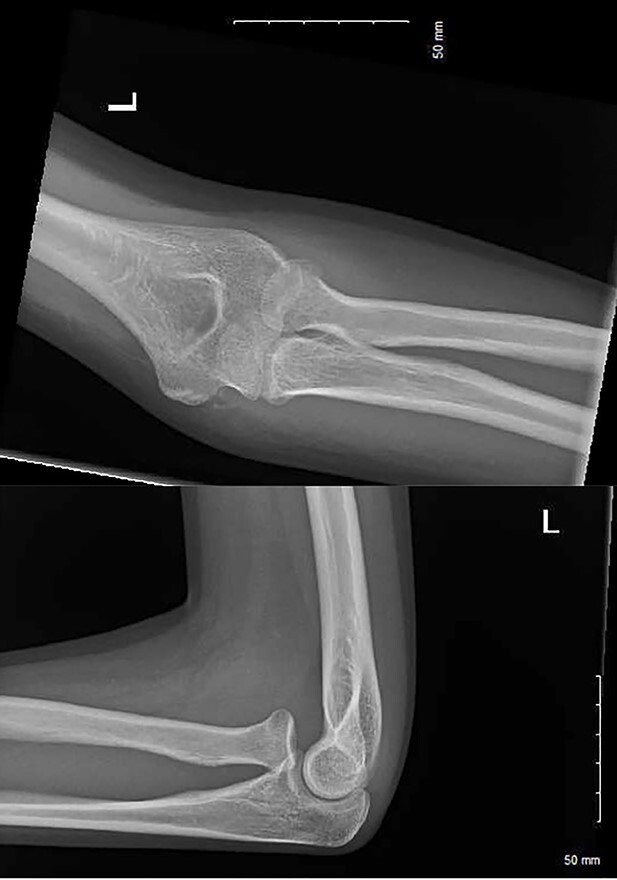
AP and lateral radiographs at 2 months post-injury.
